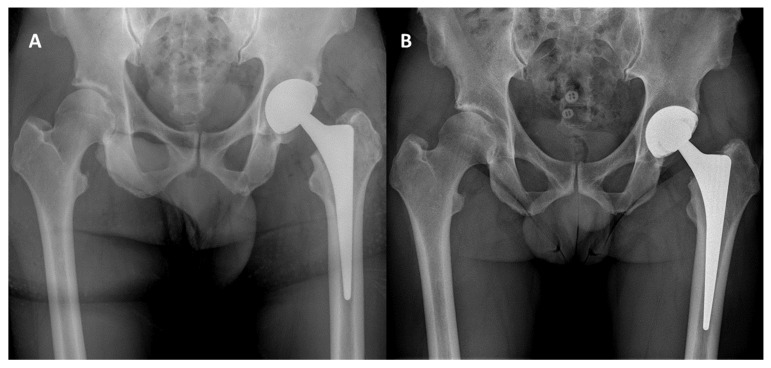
(A): Immediate postoperative radiographic control; (B): 2.5-year radiographic follow-up showing a decrease in periacetabular cancellous bone density with retention of cortical bone density.
